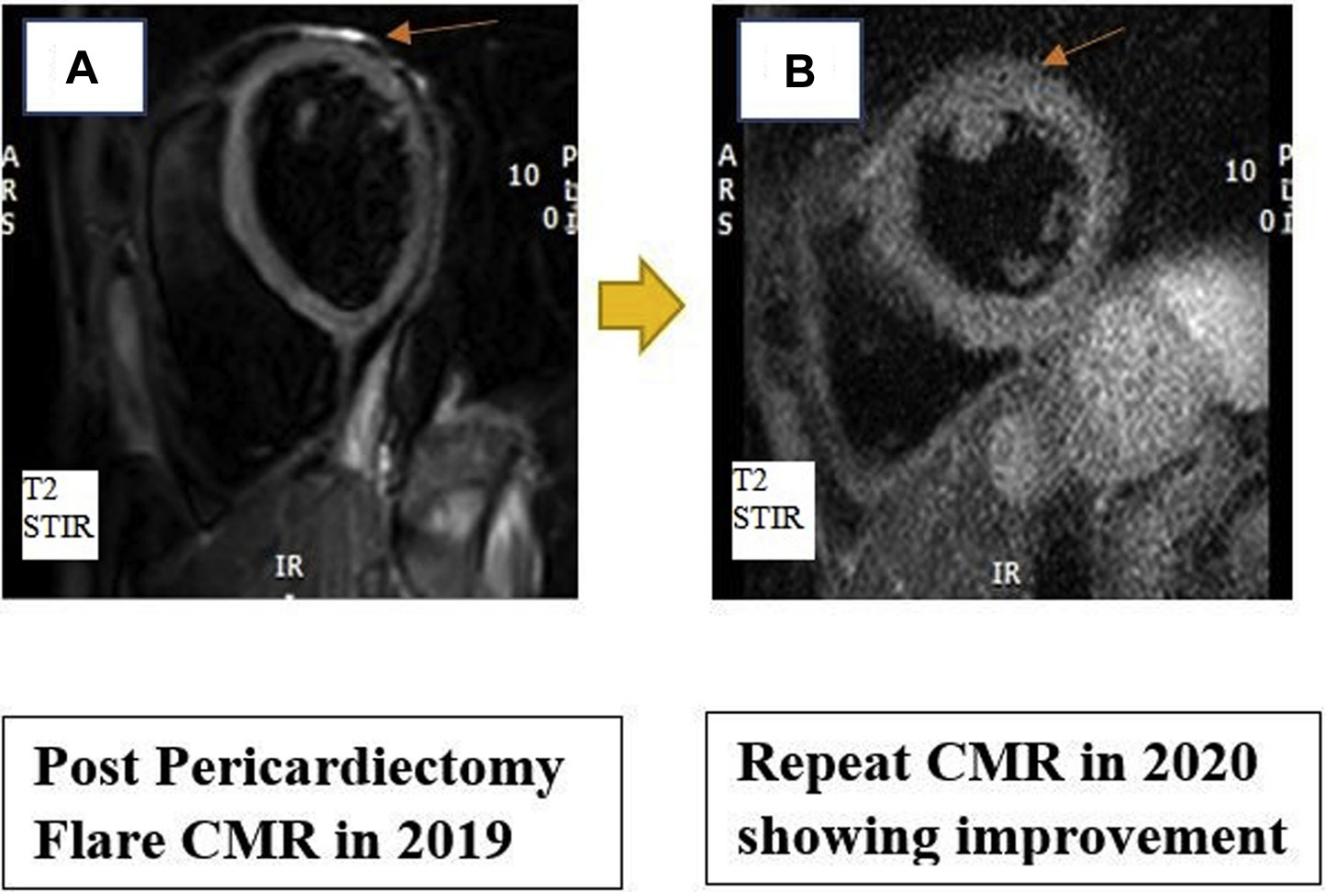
Cardiac Magnetic Resonance (A) 2019 image showing increased signal intensity on T2-weighted short T1 inversion recovery (STIR) imaging (orange arrow). (B) 2020 image showing interval improvement in signal intensity in T2-weighted STIR sequence. CMR = cardiac magnetic resonance.
